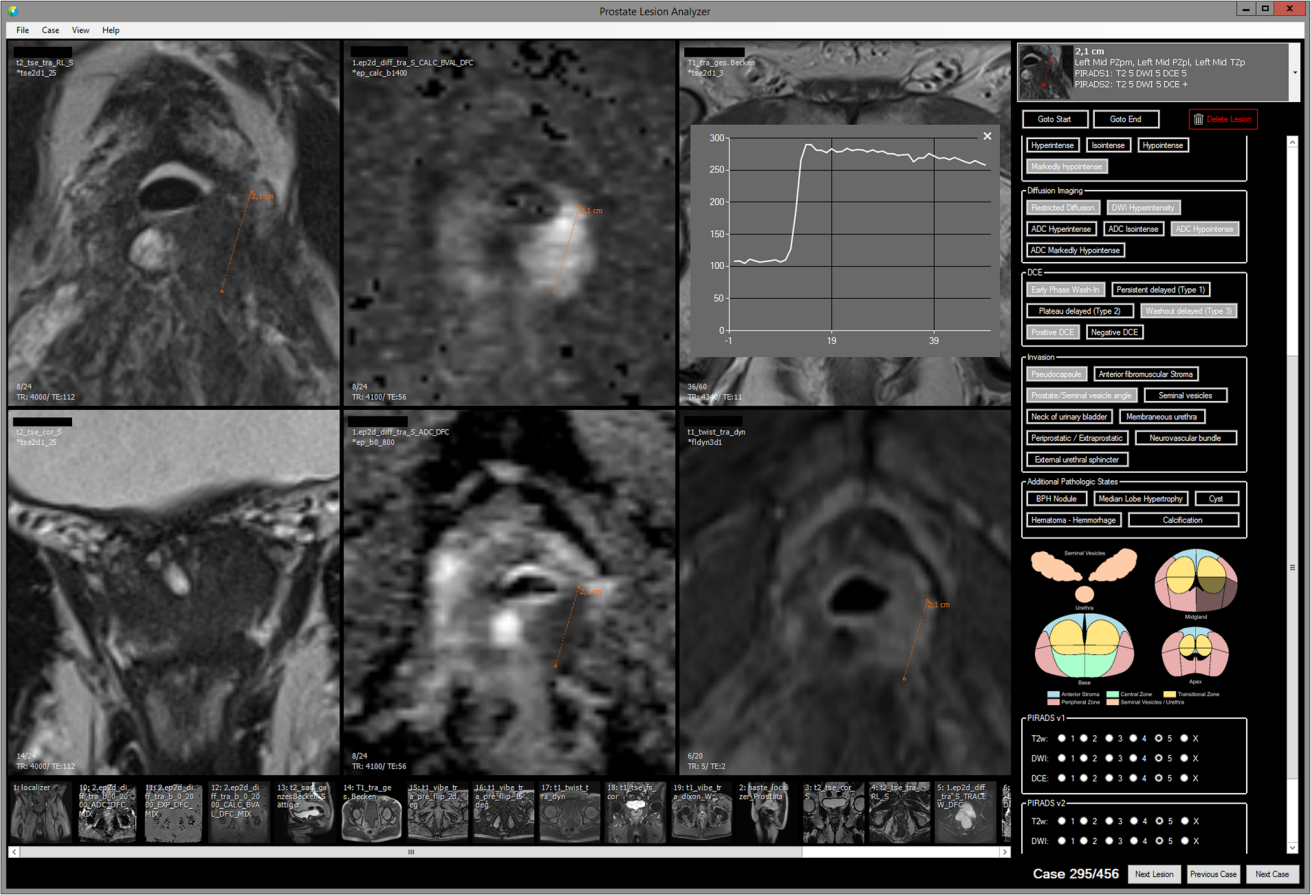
Sample screenshot of the proprietary review software Prostate Lesion Analyzer used in the study. Readers were instructed to mark lesions within the MRI pictures (left, 3 × 2 panels depicting T2WI axial, T2WI coronal, DWI/ADC, T1WI native, and DCE). Matching lexicon terms and lesion localization were selected on the panel on the right side. Definitions of lexicon terms were displayed exactly as specified in the original PI-RADS version 2 document when hovering the cursor over a term. T2WI, T2-weighted imaging; DWI/ADC, diffusion-weighted imaging/apparent diffusion coefficient; T1WI, T1-weighted imaging; DCE, dynamic contrast-enhanced imaging
